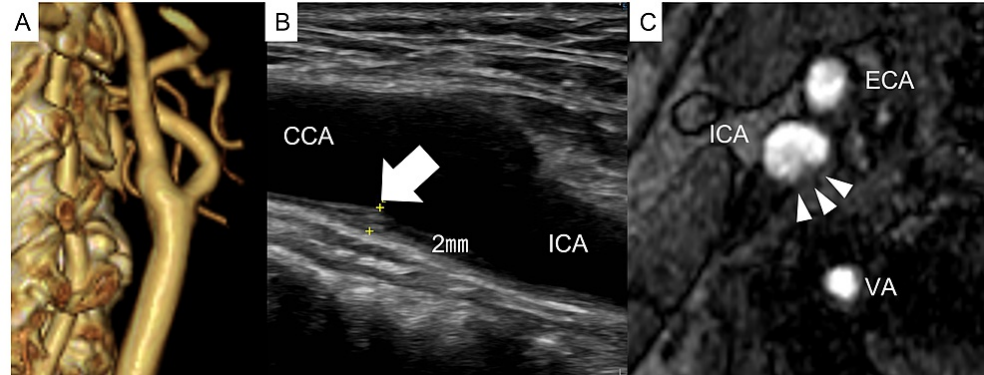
Detailed carotid artery examination after carotid free-floating thrombus dispersal A: Contrast-enhanced CT angiography showed smooth vessel wall and no stenosis; B: Carotid duplex showed a small plaque of approximately 2 mm in diameter at the bifurcation; C: Time-of-flight magnetic resonance angiography also showed a small plaque at the bifurcation. CCA: common carotid artery; ICA: internal carotid artery; ECA: external carotid artery; VA: vertebral artery.
